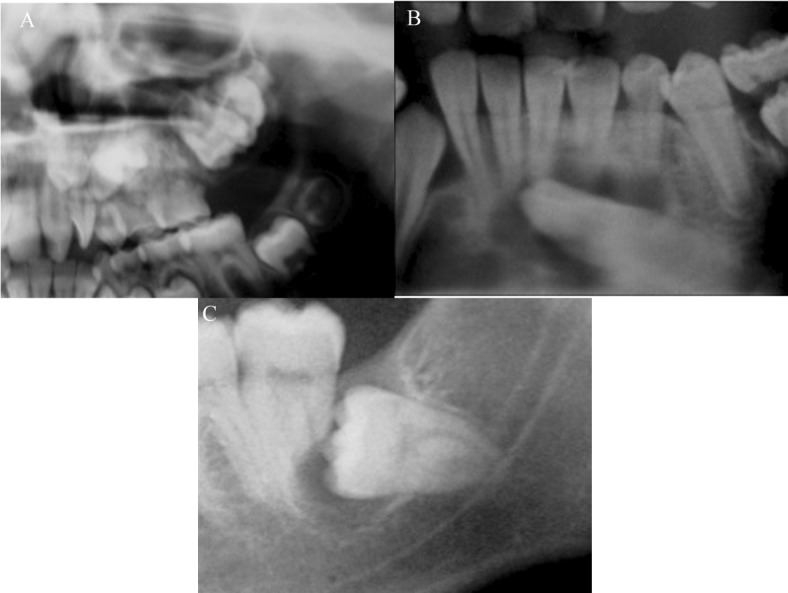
Orthopantomografic views. A) Orthopantomografic view of the case 5. B) Orthopantomografic view of the case 7. C) Orthopantomografic view of the case 8.
